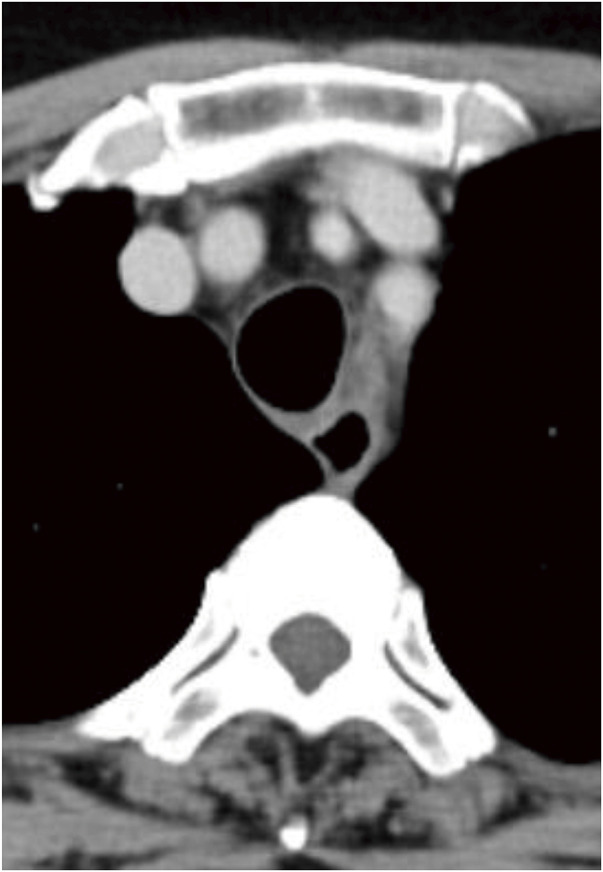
Contrast-enhanced CT at 3 months after surgery showing no evidence of recurrence.
